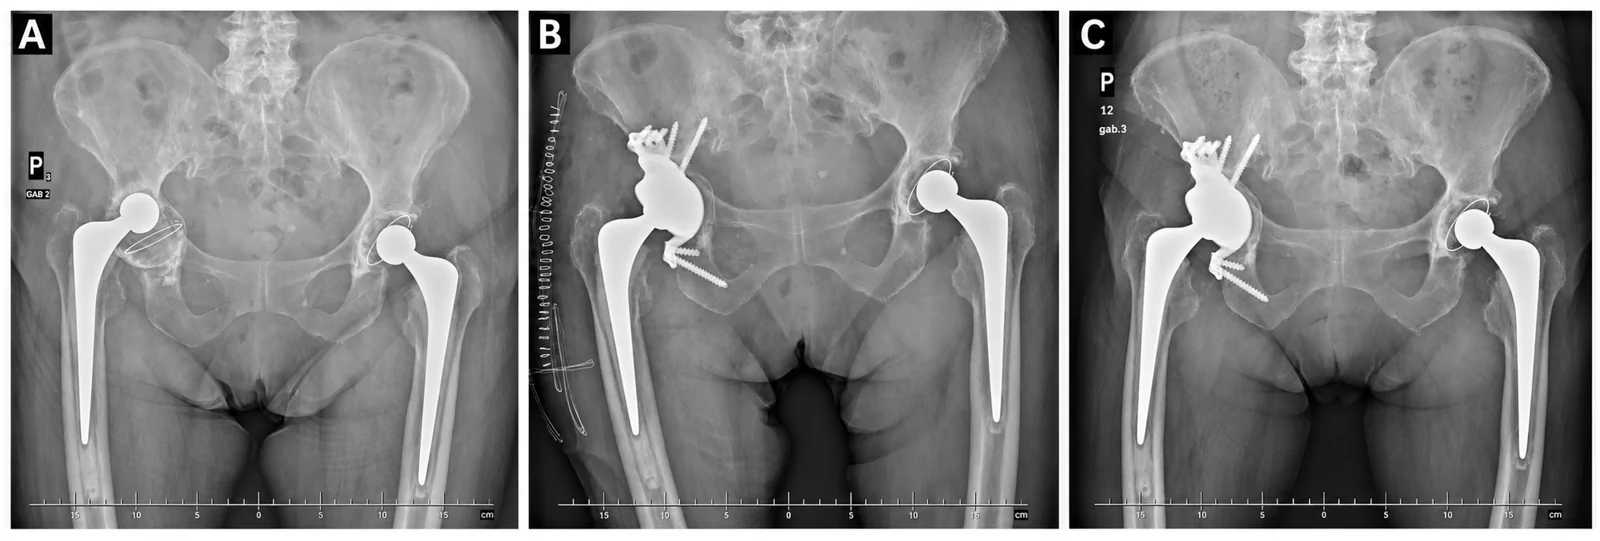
Representative case of a Paprosky type 3A acetabular defect treated with a custom-made 3D-printed acetabular implant. (A) Preoperative radiograph demonstrating prosthesis luxation with superior stem migration and extensive acetabular bone loss. (B) First day after surgery radiograph—3D custom-made reconstruction and (C) one year after surgery demonstrating stable implant position and no sign of implant subsidence or loosening.
